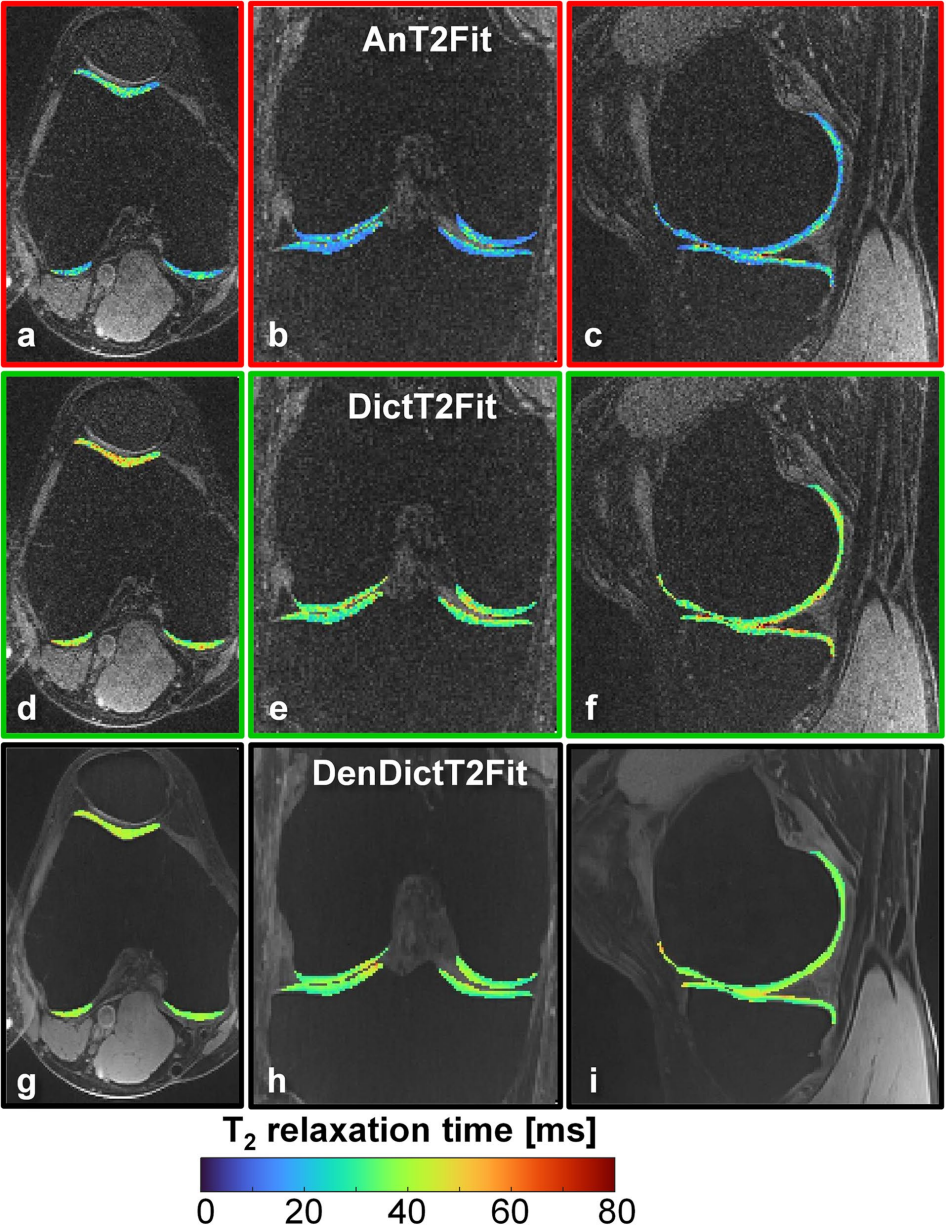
Orthogonal T2 maps of the knee of a volunteer without osteoarthritis lesions, superimposed on top of fat-suppressed T2-weighted source images. a–c Analytic maps based on standard images (AnT2Fit). d–f Dictionary-based maps of the same standard images (DictT2Fit). The T2 values are visibly more homogeneous in the different segments, while the average T2 value increased. g–i Dictionary-based maps of HD-PROST-denoised images (DenDictT2Fit). While the T2 values remain similar, the denoising is also visually apparent. *HD-PROST* High-dimensionality undersampled patch-based reconstruction
